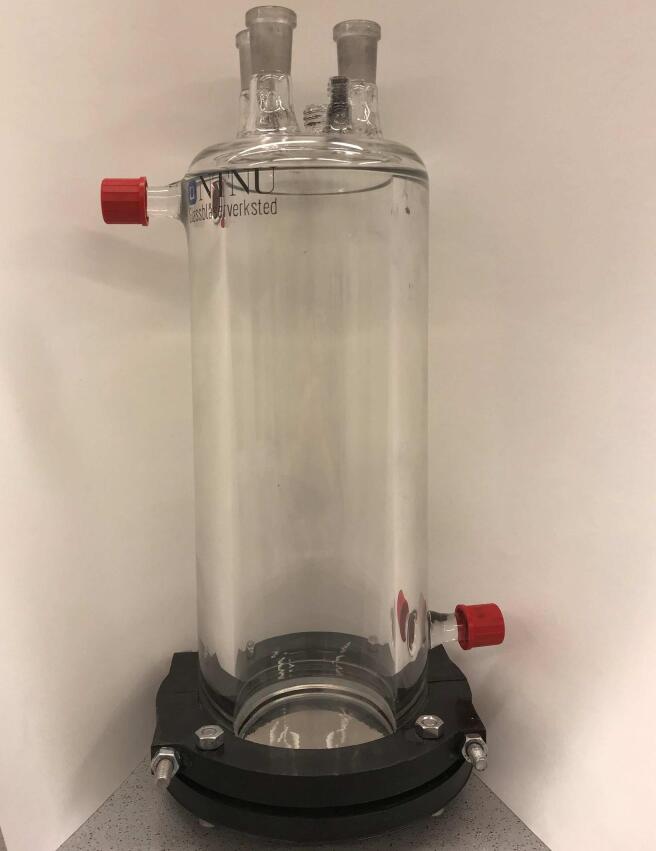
The sonochemical reactor used for all experiments. The ultrasonic transducer makes up the bottom of the reactor. Ports at the top allows for introduction of gas and sample extraction. Water can be circulated through the external cooling jacket, where the inlet and outlet are located at the bottom right and upper left, respectively.
